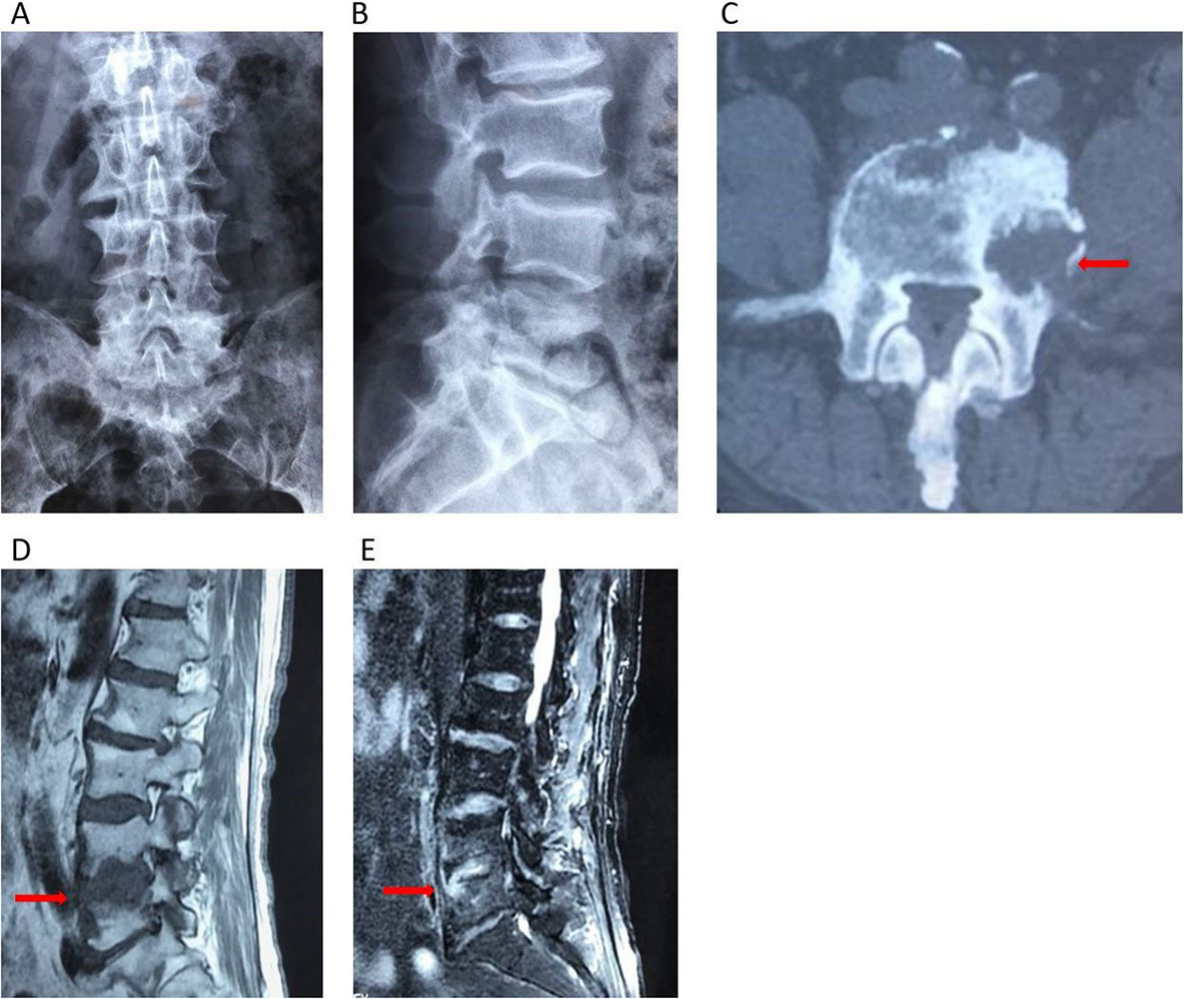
Radiological findings after 6 weeks treatment. Notes: (a and b) X-ray after treatment showed degeneration of lumbar spine, hyperplasia of vertebrae, no significant narrowing of L4–5 inter-vertebral space. (c) CT showed the area of vertebral worm-eaten-like damages were reducing and limited, the bone sclerosis appeared around the lesion (arrow).(d and e) MRI after 6 weeks treatment showed the area of inflammatory signal (hypointense on T1-WI and heterogeneous hyperintense on T2-WI) was significantly reducing (arrow)
